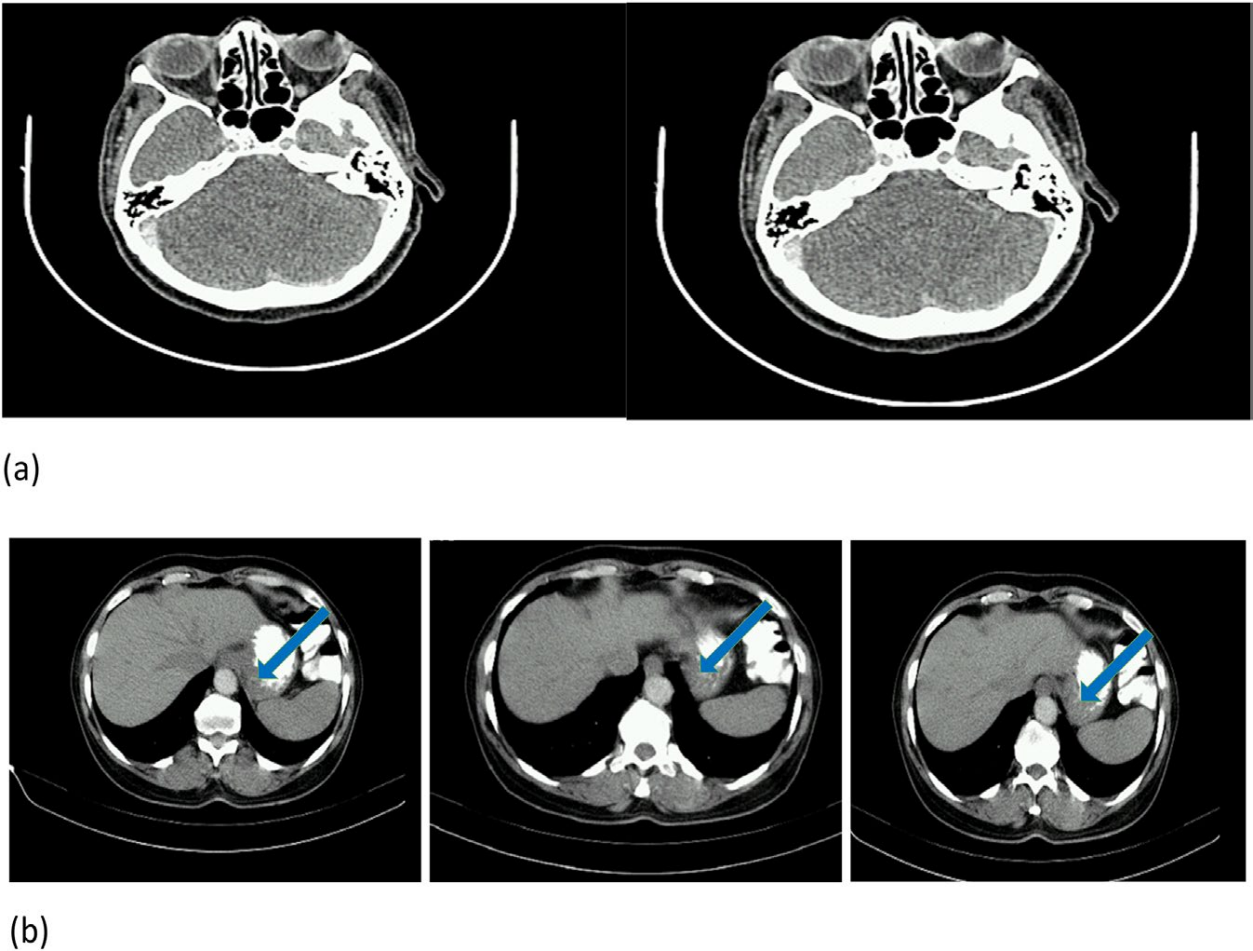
CT scan of the head and the abdomen with and without injected and oral contrast after 3 months of treatment. (A) The right orbit without lesion. (B) The blue yellow shows a slight wall thickening in fundus and cardia in the gastric.
